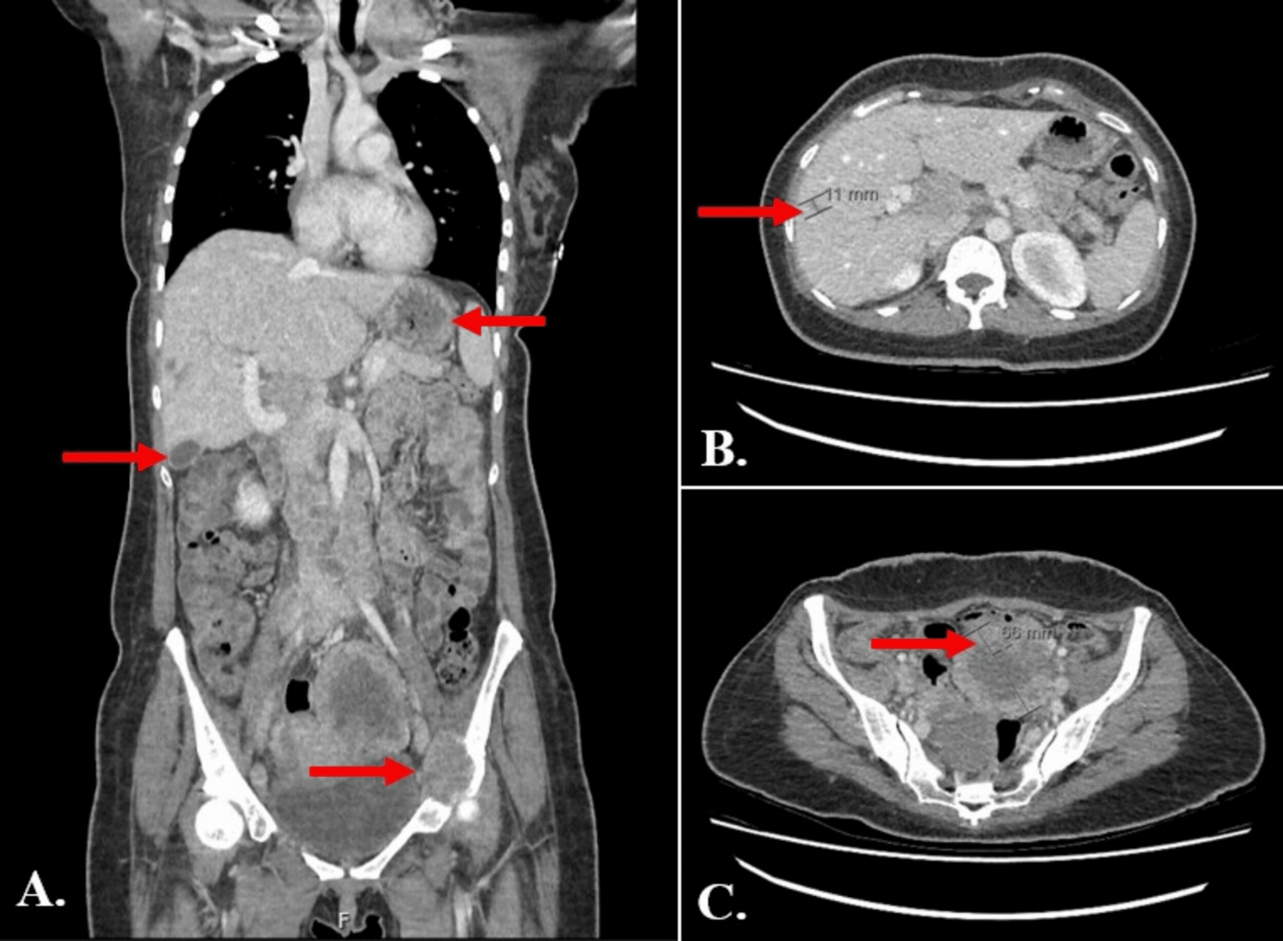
(A) Contrast-enhanced computed tomography of the thorax, abdomen, and pelvis (TAP), coronal section, showing extensive lymphadenopathy, hypoattenuating hepatic lesions, a complex left ovarian cystic mass, and a lytic lesion involving the left acetabular roof (arrows). (B-C) Axial sections demonstrating a small hypoattenuating hepatic lesion (11 mm; arrow) and a left ovarian cyst (6.6 cm) with nodular enhancing components (arrow).
